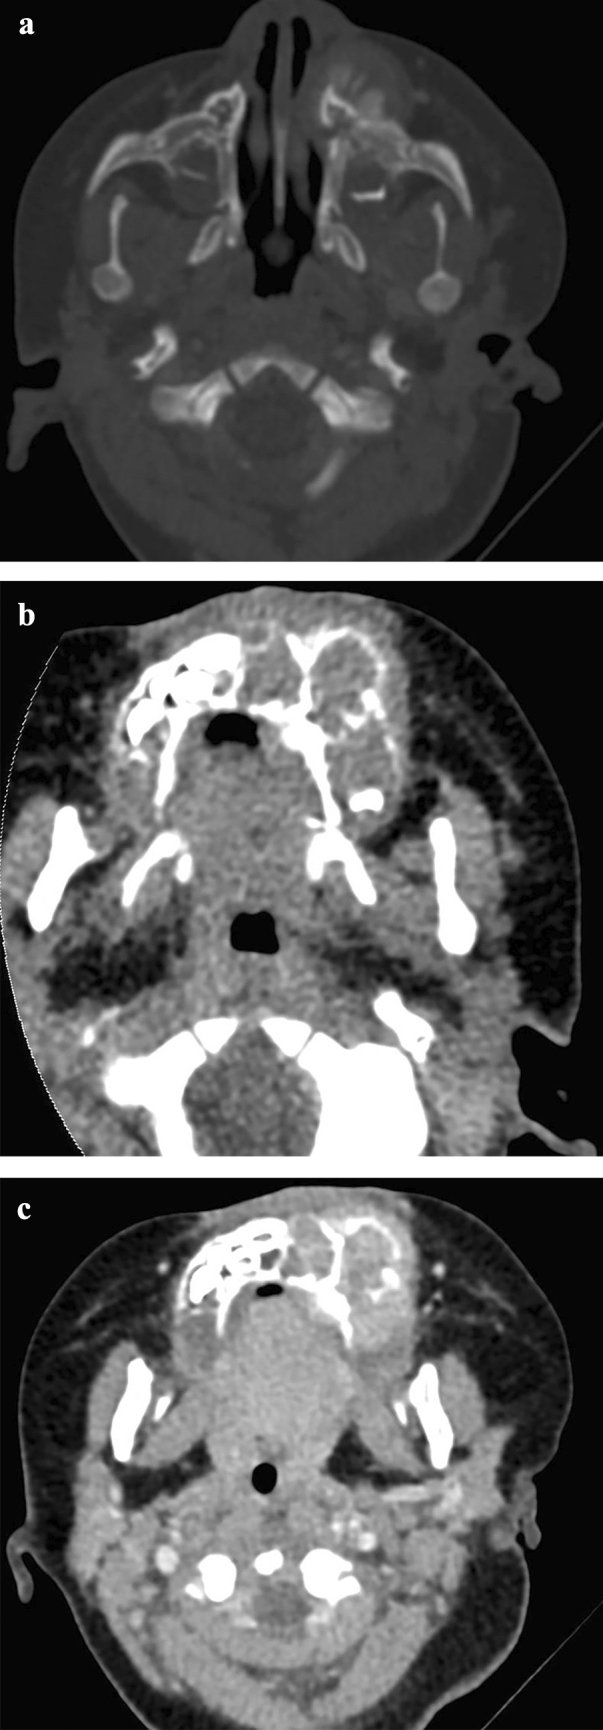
Case 2. a/b axial CT images revealed osteolytic and expansive bone destruction of left maxilla, involving all maxillary sinus walls. Spiculated/ sunburst periosteal reaction was obvious. Irregular and ill-defined soft-tissue mass in which tooth germs floated was noticed in the bone destruction area. c axial contrast-enhanced CT scans showed medium heterogeneous enhancement of the soft-tissue mass combining with liquefactive necrosis areas
